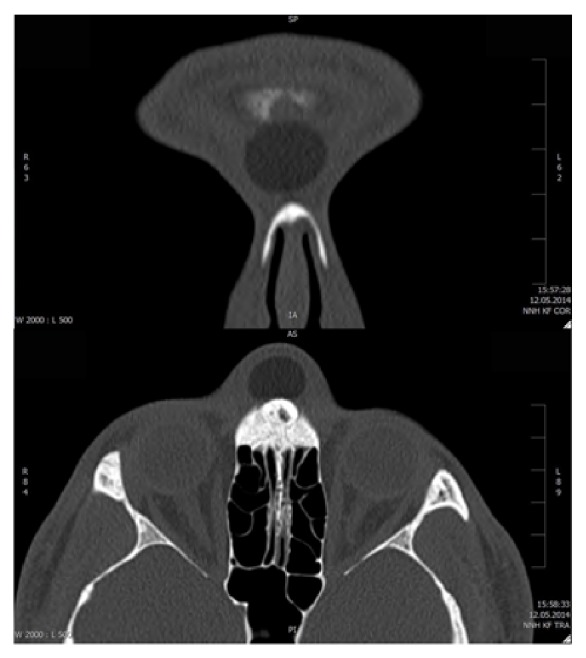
Coronal and axial CT images of the mass.
